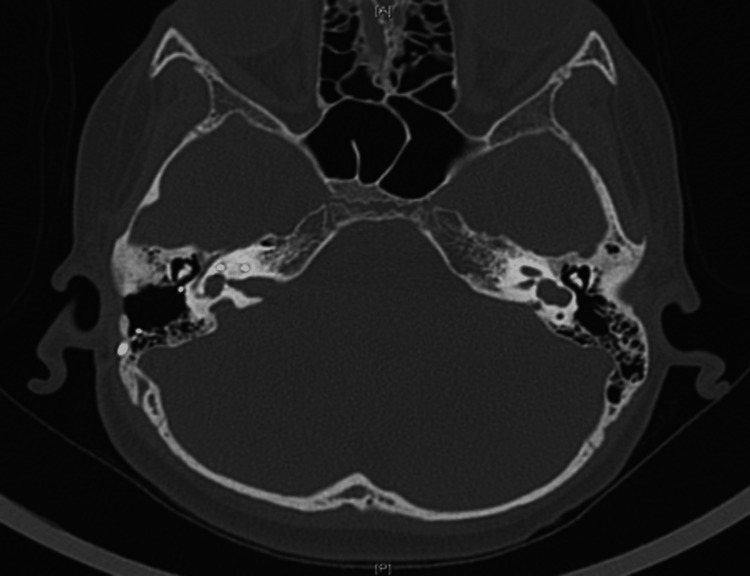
CT temporal bone axial cut showing near-complete right labyrinthine ossification around the electrode; CI electrode is intact within the cochlea. On the left, there is severe stenosis of the lateral and atretic medial part of left internal auditory canal. CI, cochlear implant
